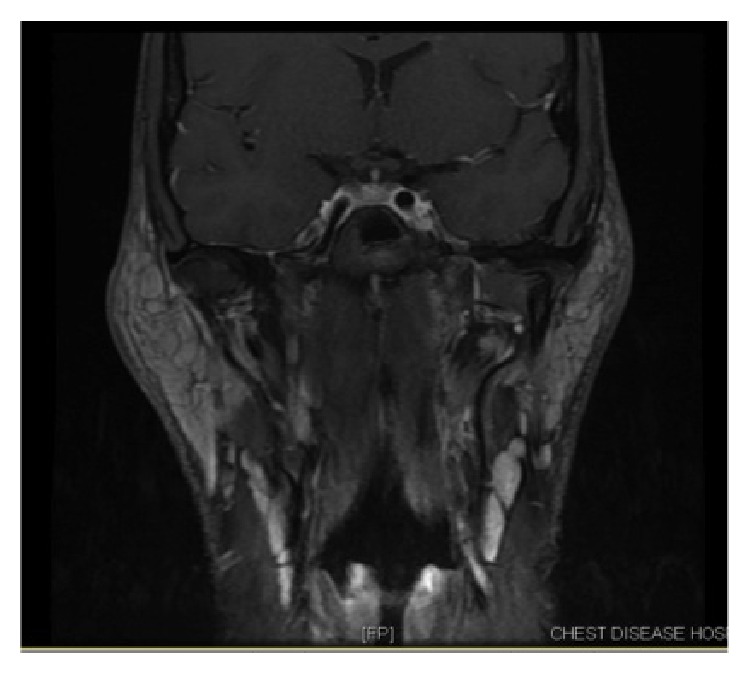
Preoperative MRI T1-weighted coronal cut: bilateral enlarged parotids and intraglandular lymph nodes.
